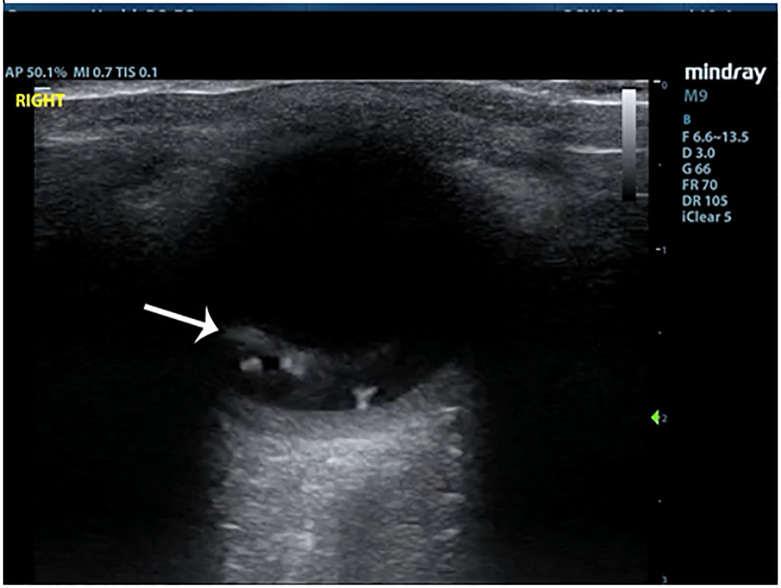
B-mode ultrasound of the right eye showing hyperechoic band (arrow) in the posterior segment with underlying scattered echoes.
